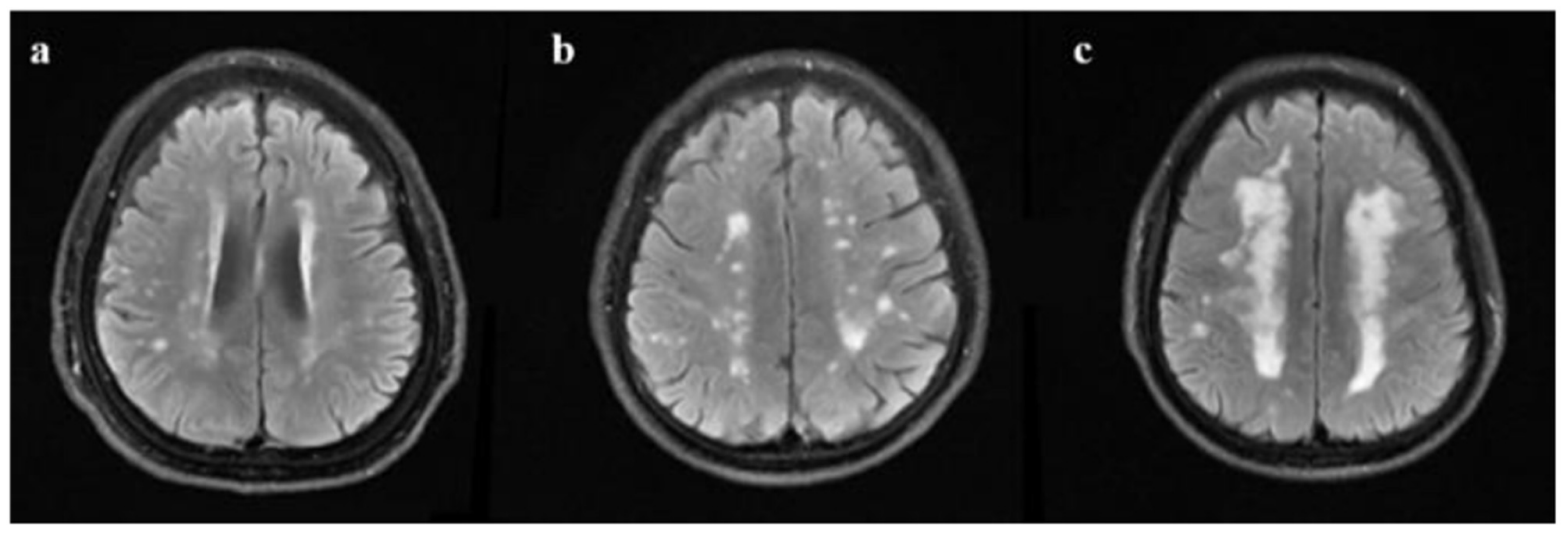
Fazekas scale scores of deep white matter hyperintensities. (a) punctate foci; (b) beginning confluence of foci; (c) large confluent areas (instances of T2 FLAIR from patients with aCSVD).
